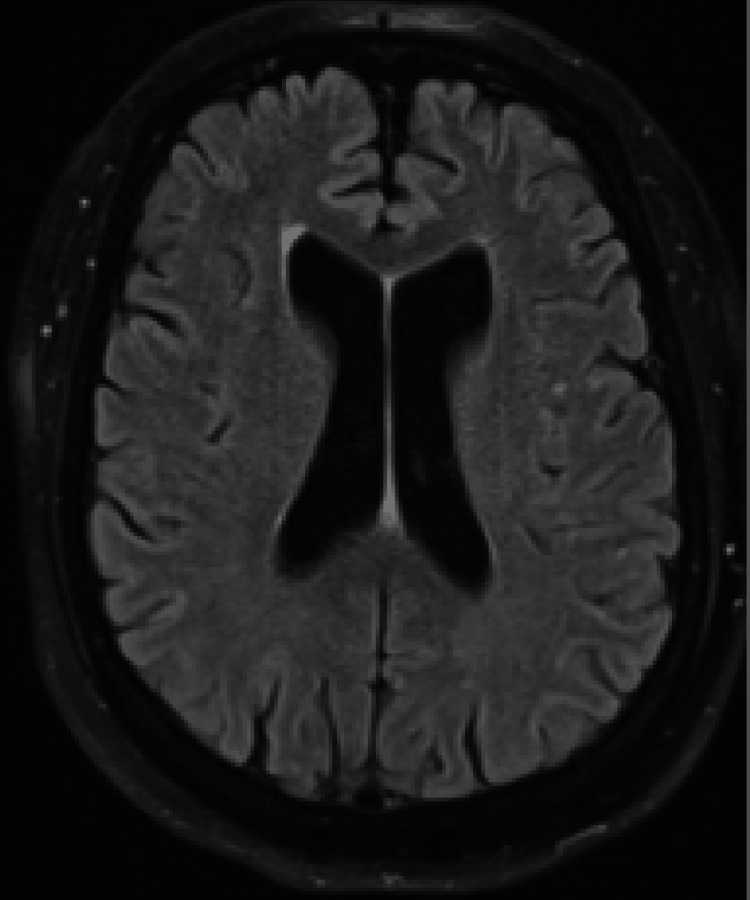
MRI of the brain is unremarkable
